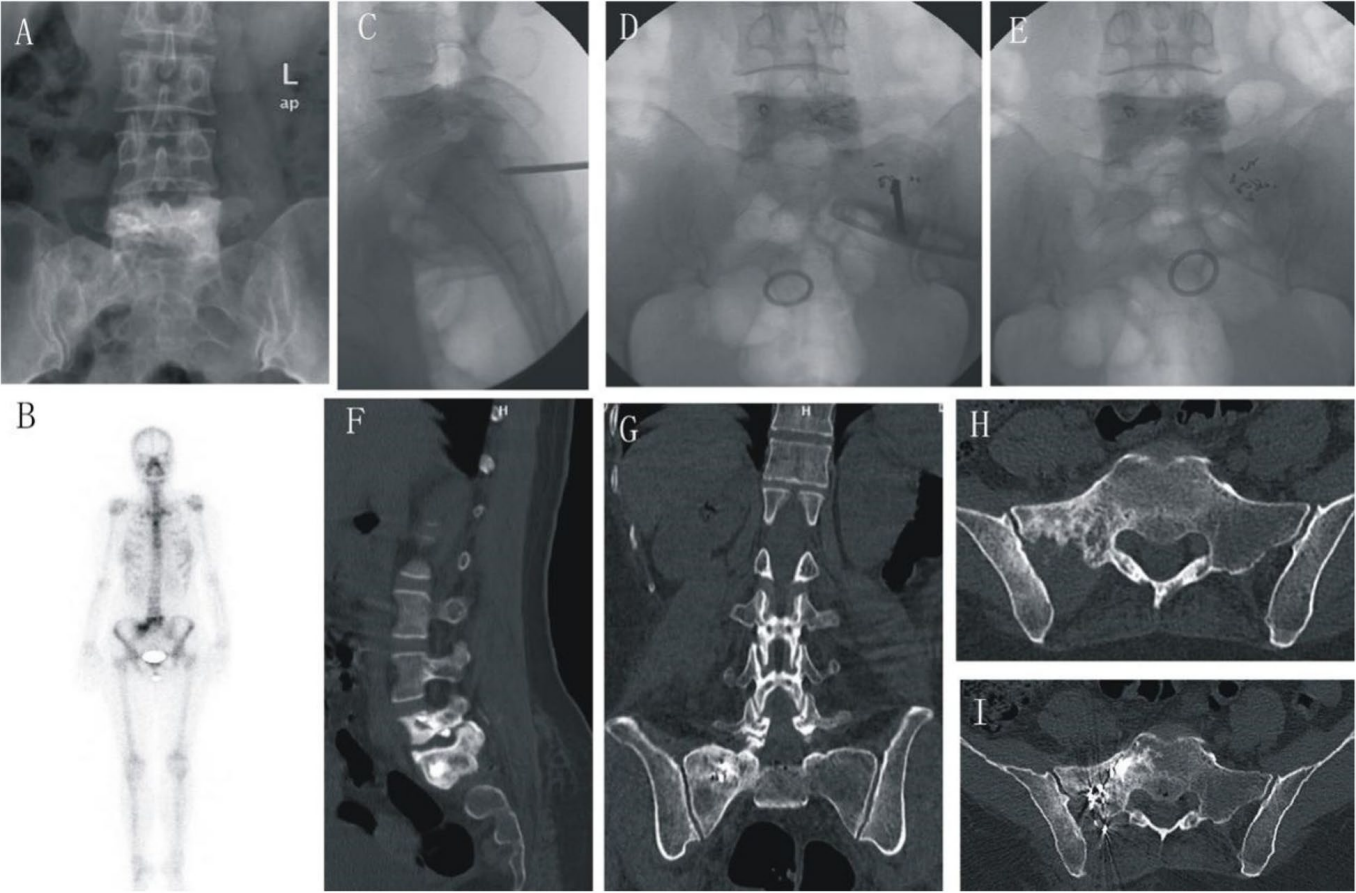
Female patients, 4 years after radical resection of the right lung, L5PVP combined with ^125^I seed 3 years after implantation, Preoperative VAS score 8.7 points. A Preoperative DR, CT shows that the right side of the block of S1 vertebrae and the adjacent vertebrae, and right iliac bone increased in inhomogeneity; B: SPECT: To the right sacroiliac joint abnormalities metabolically active, more consideration transferred. C–E S1PVP combined with ^125^I seed implantation. F–I Postoperative CT showed bone cement and ^125^I particle distribution in the lesion area, without leakage of bone cement. Postoperative VAS score 2.7 points, significant pain relief
